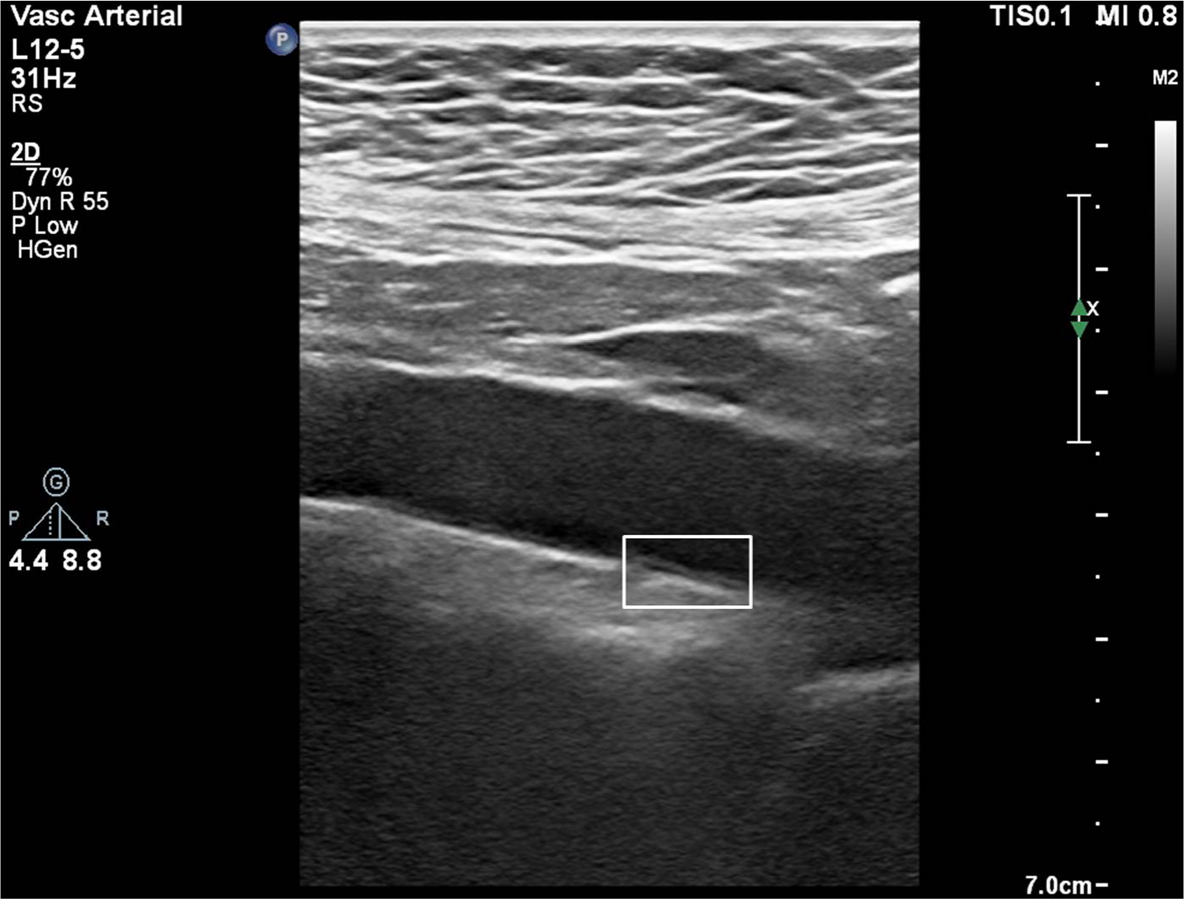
Aortic IMT measurements with B-mode ultrasound from the level of renal artery bifurcation to iliac artery bifurcation
